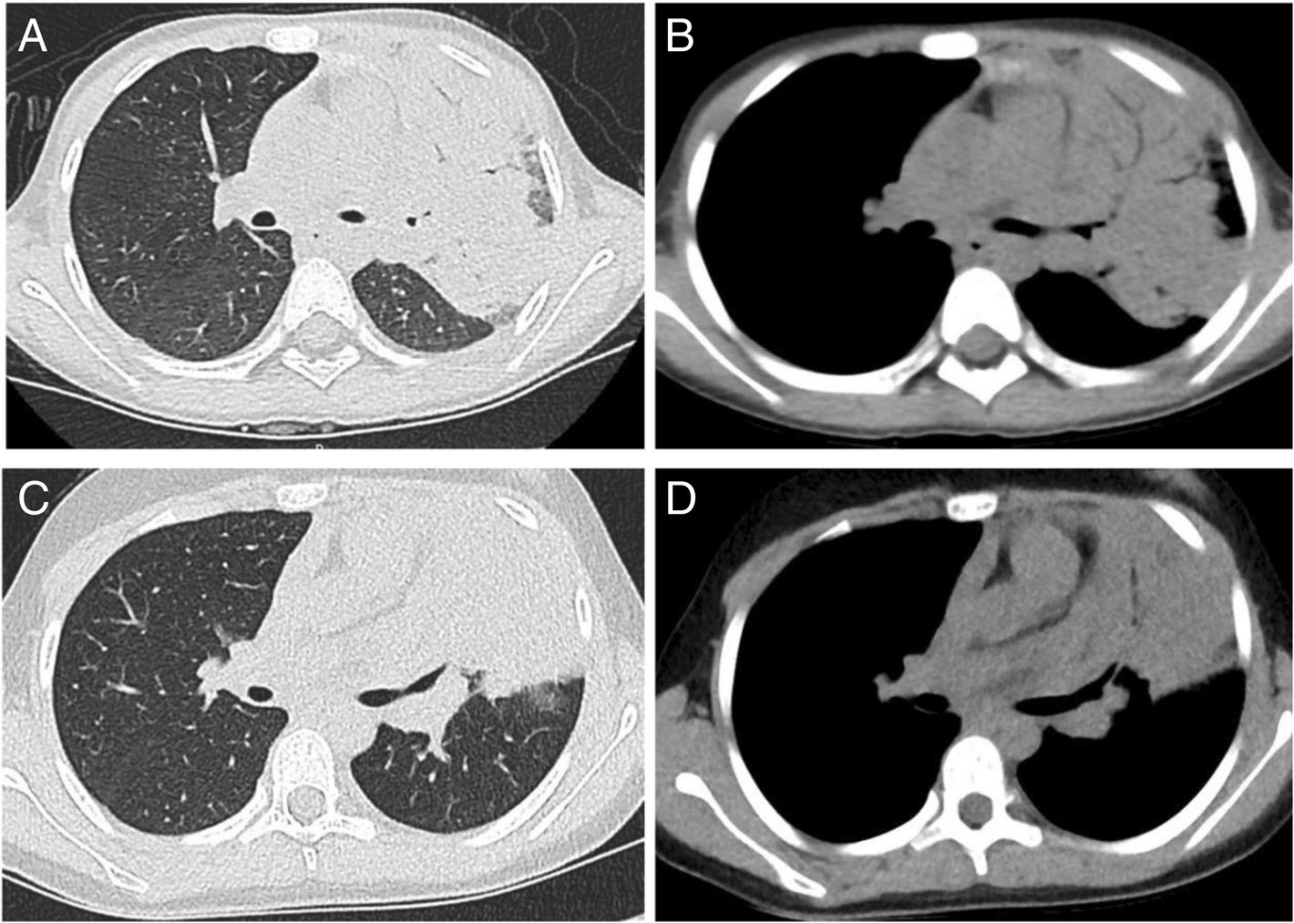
Examples of CT images from two patients with tuberculosis and pneumonia. (a) and (b) show the lung window and mediastinal window of the axial CT image of a 7-year-old girl with pulmonary TB in the left upper lobe. (c) and (d) show the lung window and mediastinal window of the axial CT image of a 10-year-old girl with CAP in the left upper lobe
